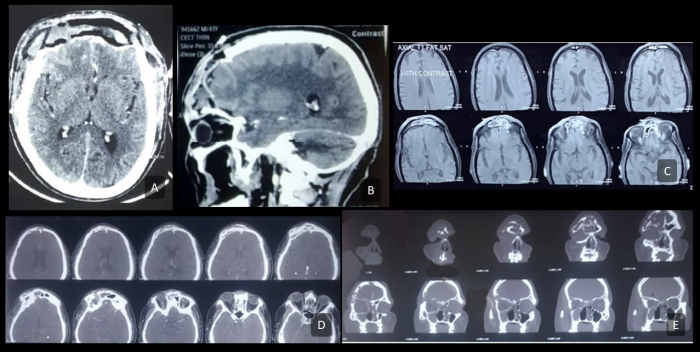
Pre-operative Imaging. A and B: Axial and sagittal contrast-enhanced CT, respectively, showing complete erosion of frontal bone with necrotic soft tissue debris between the skin and dura; the dura appears enhanced. C: T2-weighted axial MRI cuts depicting heterogeneously enhancing soft tissue density in the frontal sinus with posterior table erosion. D and E: Axial and coronal contrast-enhanced CT cuts, respectively, depicting the erosion of anterior and posterior tables of frontal sinus, suggestive of osteomyelitis.
